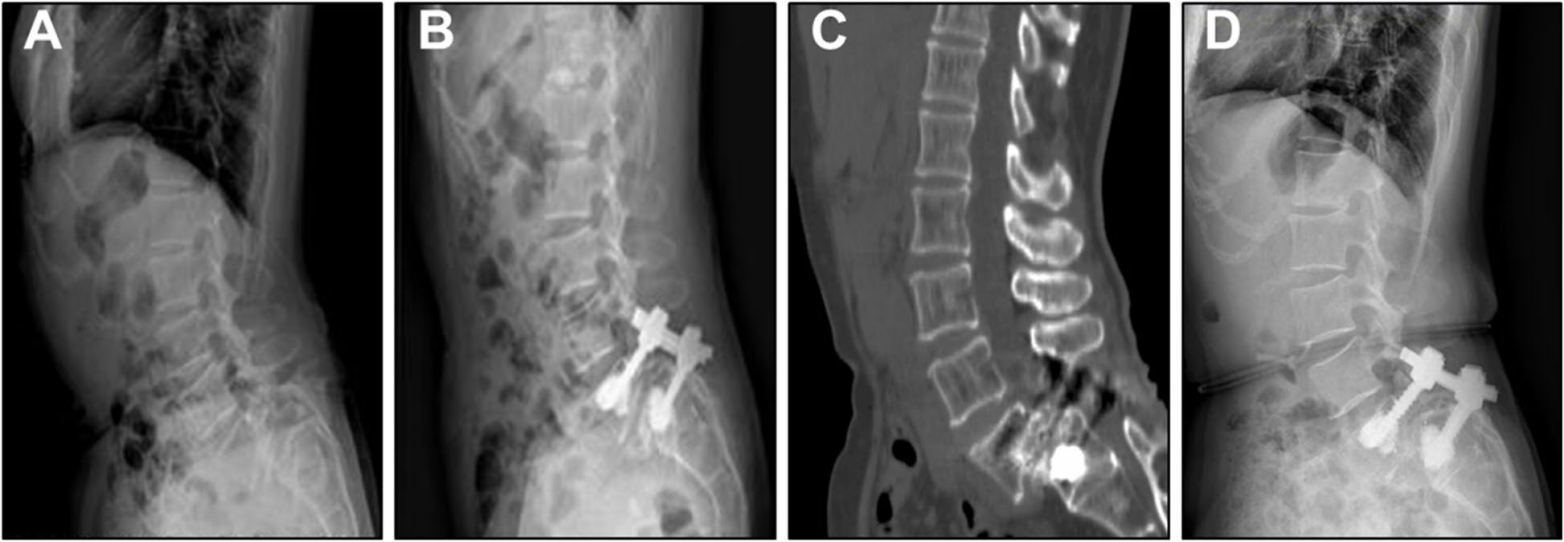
A 54 years old female, BMD -2.8SD, treated with CICPS fixation by PMMA augmentation. A Preoperative radiographs showed L5 vertebral body was of level IV forward slip, spondylolysis type. B X-ray examination showed good vertebral reduction and no leakage of bone cement one week after operation. C CT results showed good fusion 36 months after surgery. D 60 months after surgery, no obvious double ring sign, no loosening, no fracture or pulling out was observed
